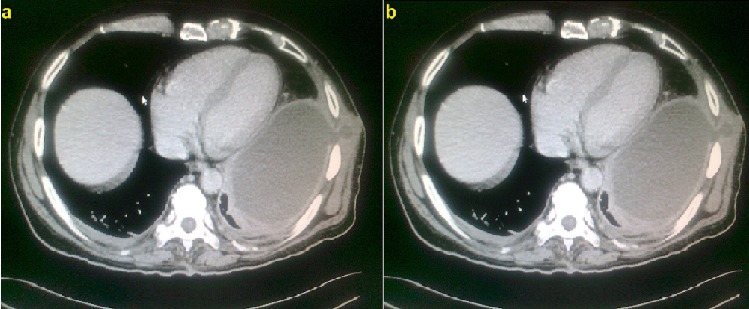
(a and b) CT scan chest showing left sided chronic empyema
